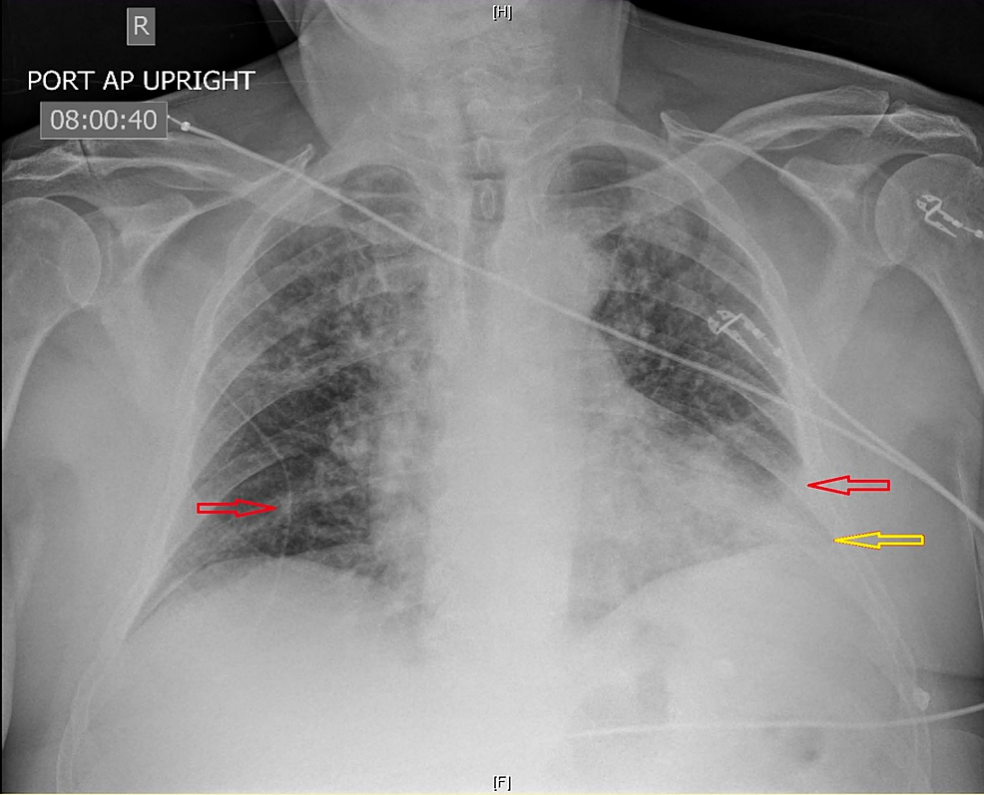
Chest X-ray showing bilateral basal patchy opacities (red arrows) and left-sided pleural effusion (yellow arrow).
